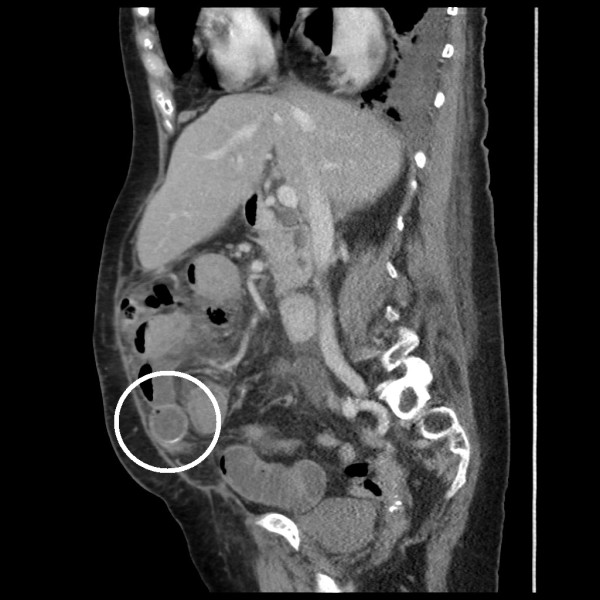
Sagittal computed tomography image shows a calculus in the distal small bowel with proximal dilatation.
